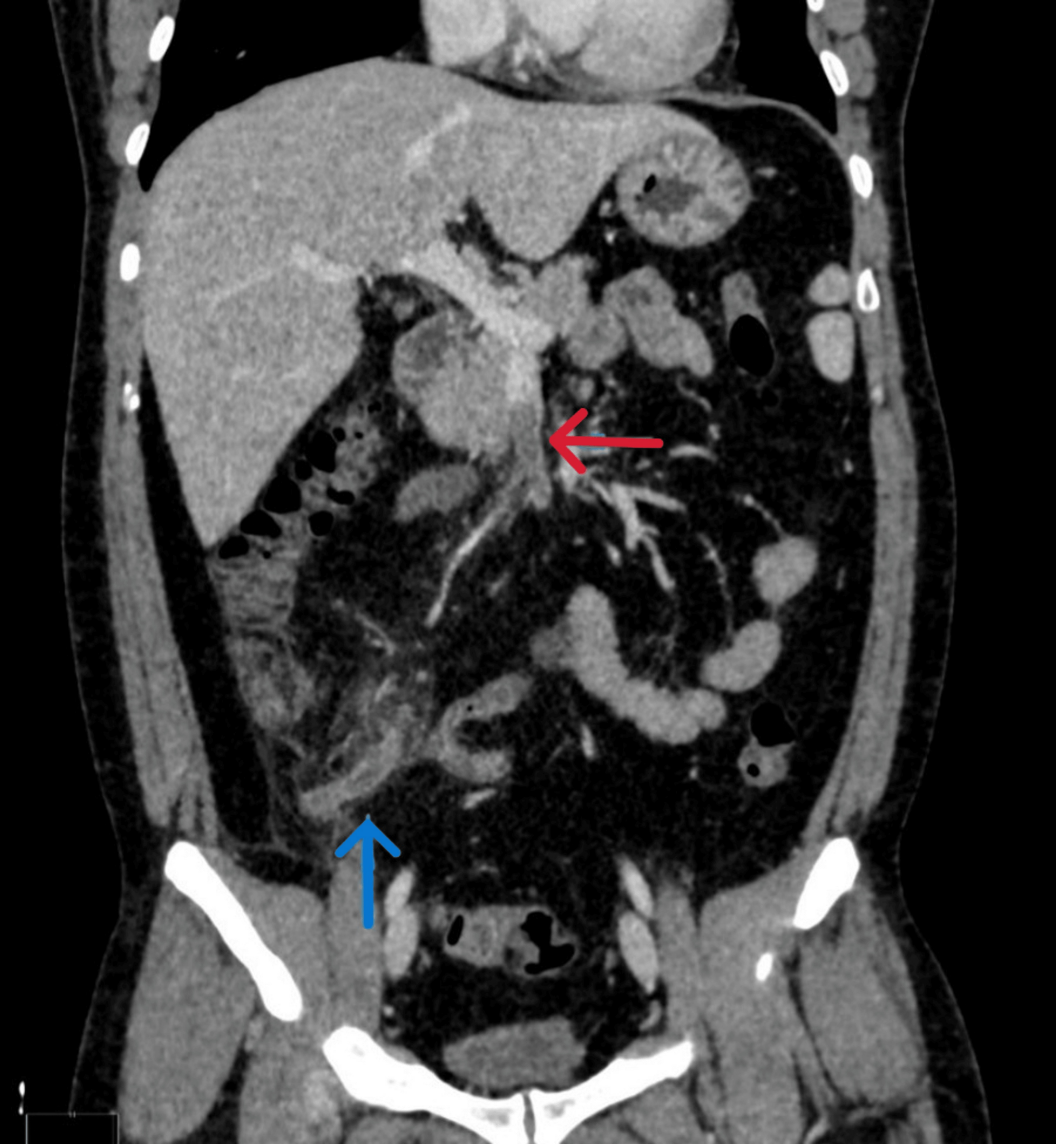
Coronal CT abdomen showing concurrent SMVT and acute appendicitis A CT scan of the abdomen in coronal view demonstrates a filling defect in the SMV, consistent with venous thrombosis (red arrow). The appendix appears inflamed with a thickened wall, suggestive of acute appendicitis (blue arrow). SMV, superior mesenteric vein; SMVT, superior mesenteric vein thrombosis
